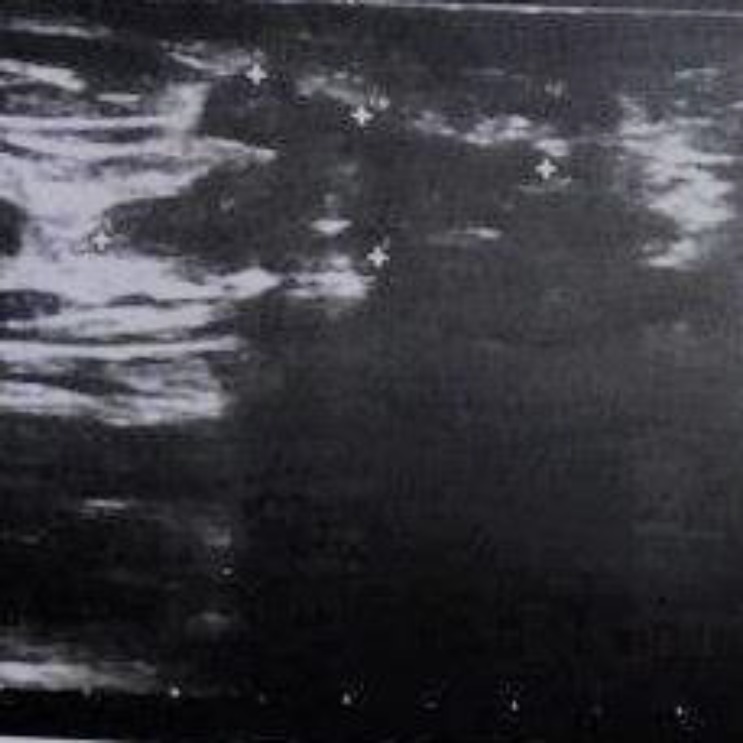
Ultrasonography image shows a 26x12x25mm vascularized, hypoechoic, irregular in shape lesion in the abdominal wall, 7mm deep to the skin
